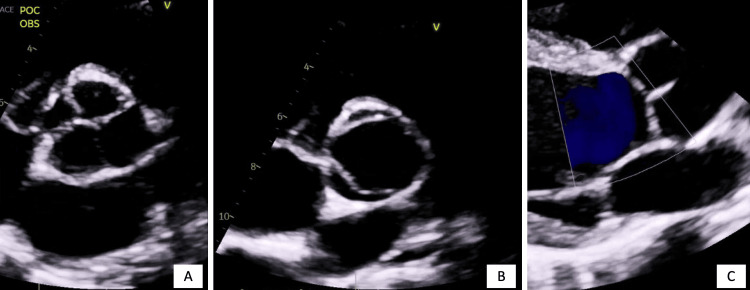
Quadricuspid Aortic Valve Imaging Panel A shows transthoracic echocardiography demonstrating the quadricuspid aortic valve in a closed position with leaflets approximated, in the parasternal short axis view; note the leaflet sizes with one large cusp, two equally sized cusps, and one small cusp, indicative of Hurwitz and Roberts class D. Panel B demonstrates the quadricuspid aortic valve in the open position in the parasternal short axis view. Interestingly, in this view the valve morphology appears bicuspid. Panel C demonstrates the quadricuspid aortic valve parasternal long axis view, demonstrating the lack of aortic regurgitation, evidencing appropriate approximation of the valve leaflets upon closure.
